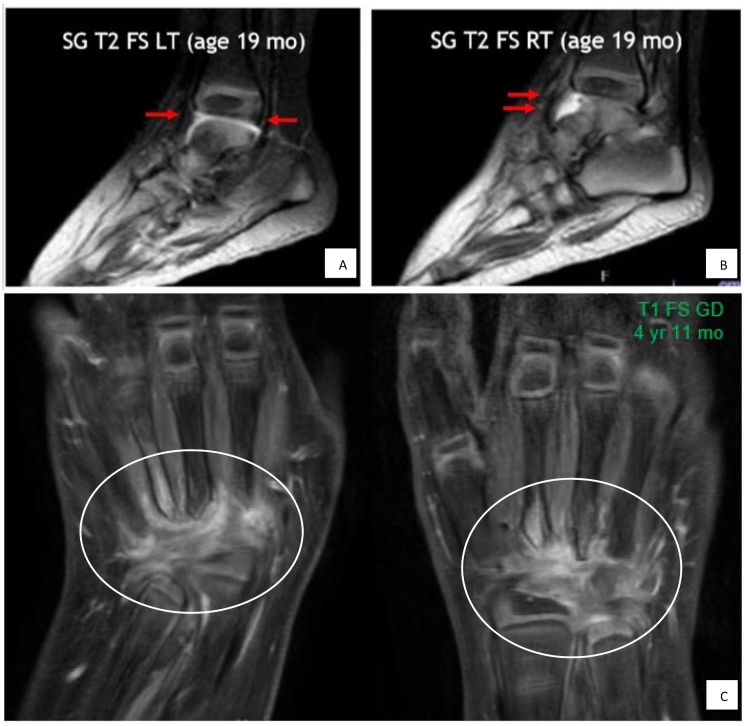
Joint inflammation on MRI. A, B: Presence of joint effusions and synovitis in bilateral ankle compartments at age 19 months. These images are prior to initiation of treatment with anti-rheumatic agents. C: Redemonstration of diffuse inflammation in bilateral wrists after discontinuation of methotrexate therapy.
